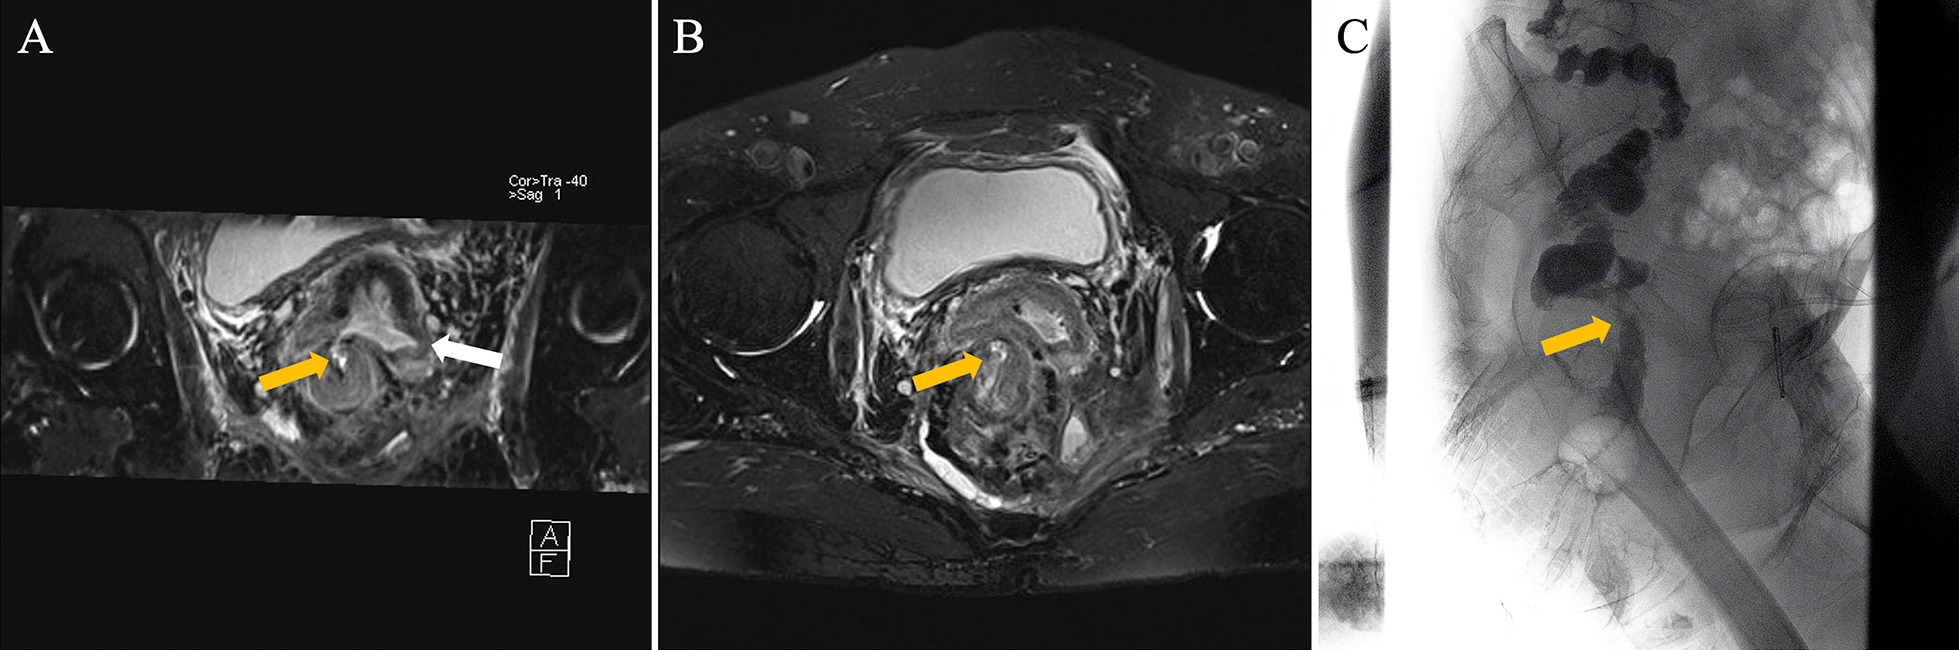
Oblique coronal (A) and axial (B) (fat suppression) T2-weighted MR images clearly show a fistula tract (yellow arrow) and an abscess (white arrow) in a 70-year-old woman. Barium enema (C) shows the unclear course of the fistula tract (yellow arrow)
